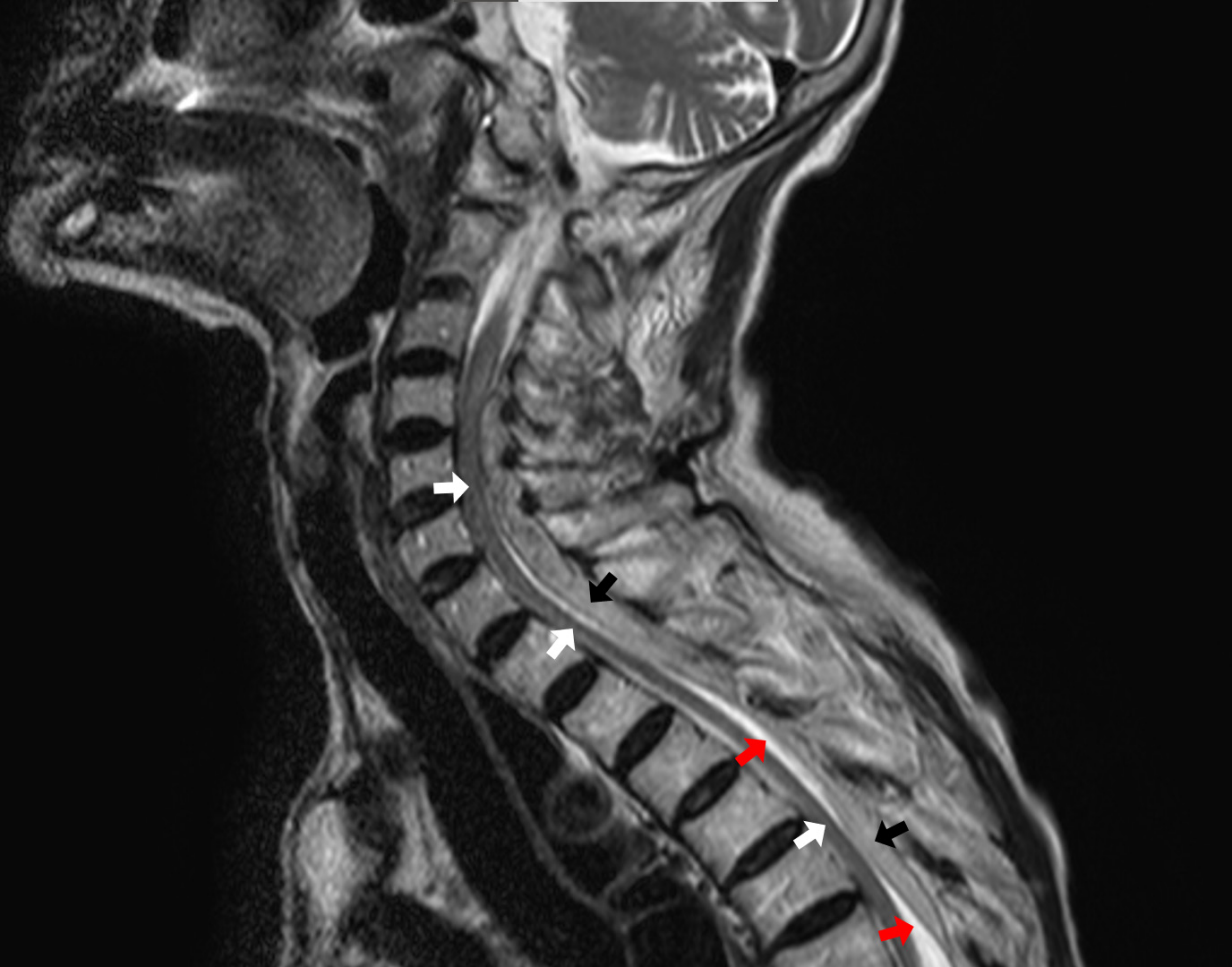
Sagital view on T2‐weighted imaging of MRI revealed a three‐layered structure in the spinal canal, consisting of the spinal cord (white arrows), spinal fluid (red arrows), and hematoma (black arrows).
